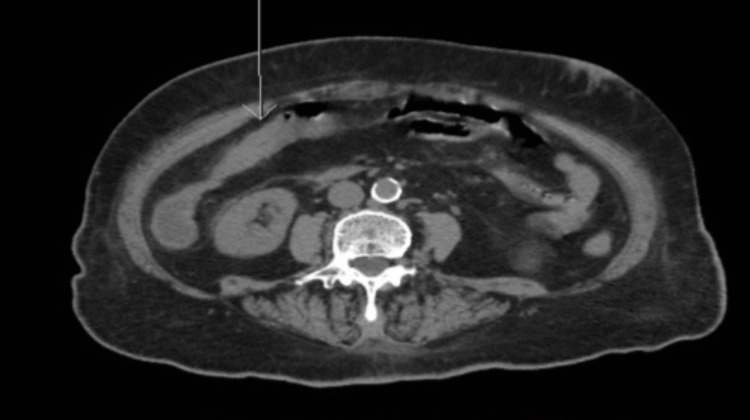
CT angiogram of abdomen demonstrating a focus of thickened and friable intestinal wall in the proximal transverse colon with minimal fatty stranding, indicating ischemia or inflammation.
